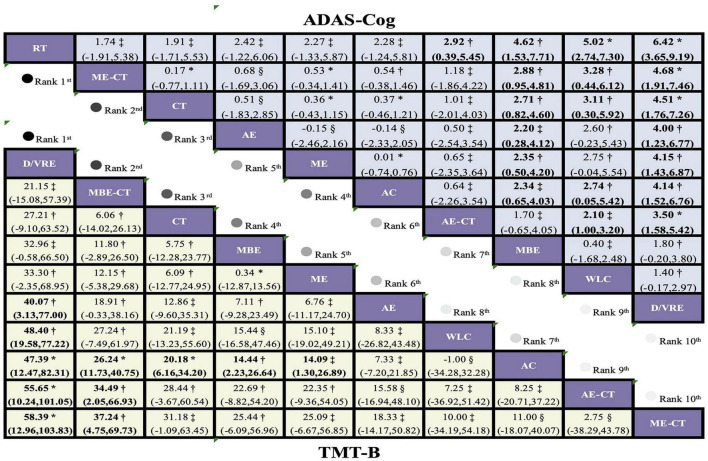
League table from the network meta-analysis comparing intervention nodes in older adults with mild cognitive impairment. Mean differences (MDs) with 95% confidence intervals (CIs) are presented for TMT-B in the lower-left triangle and ADAS-Cog in the upper-right triangle. Symbols denote certainty of evidence according to the GRADE approach: * high; † moderate; ‡ low; § very low. AE, aerobic exercise; RT, resistance training; MBE, mind–body exercise; ME, multicomponent exercise; D/VRE, digital or virtual reality–based exercise; CT, cognitive training; AE-CT, aerobic exercise plus cognitive training; RT-CT, resistance training plus cognitive training; MBE-CT, mind–body exercise plus cognitive training; ME-CT, multicomponent exercise plus cognitive training.
